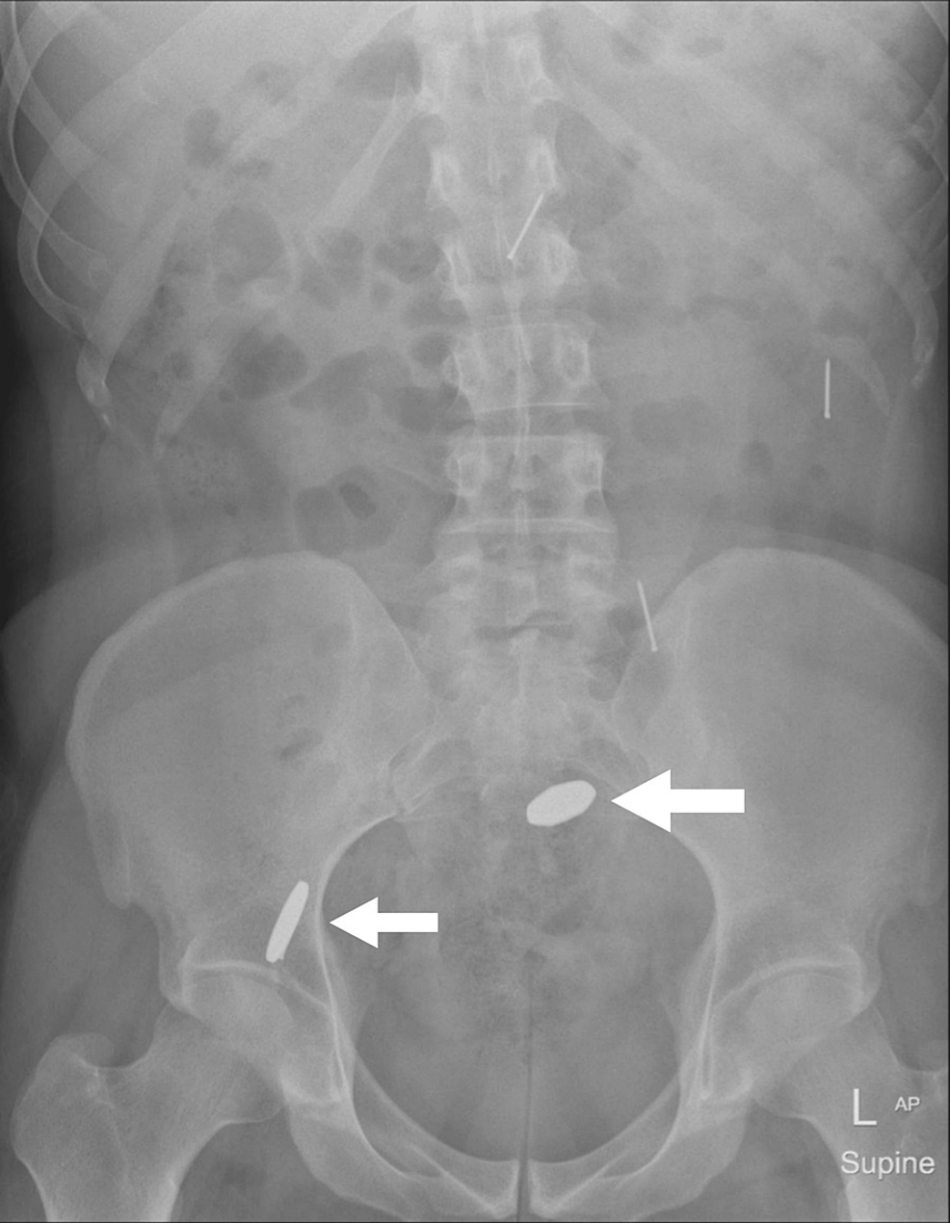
Abdominal X-ray showing two new coins.
